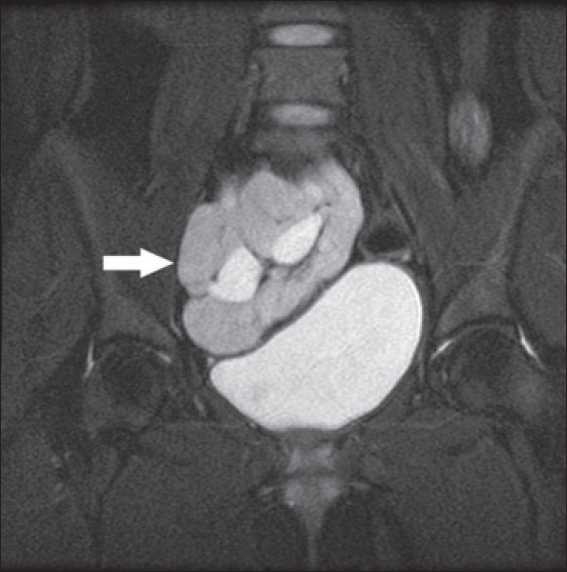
MRI showing pelvic kidney in relation to bladder
